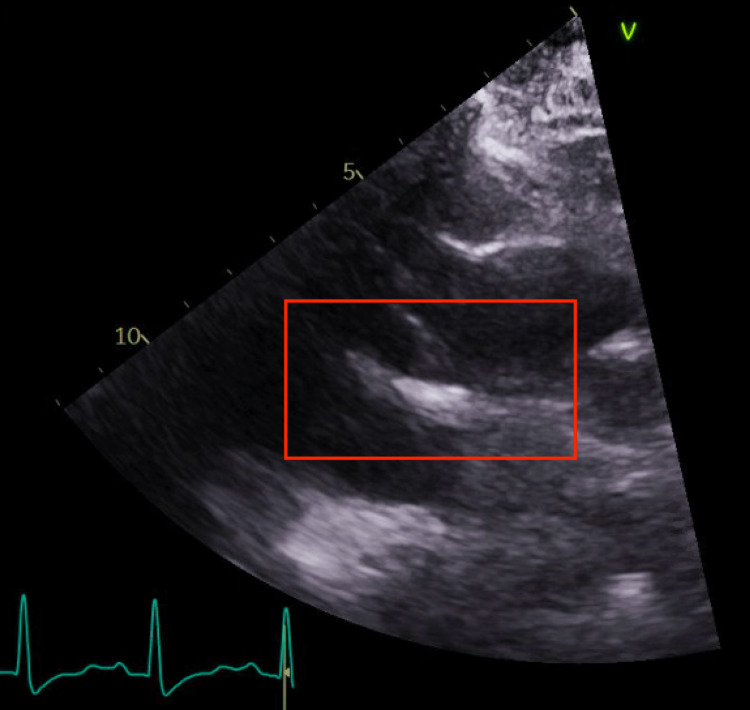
Transthoracic echocardiogram revealing a mobile linear echodensity in the aortic root
